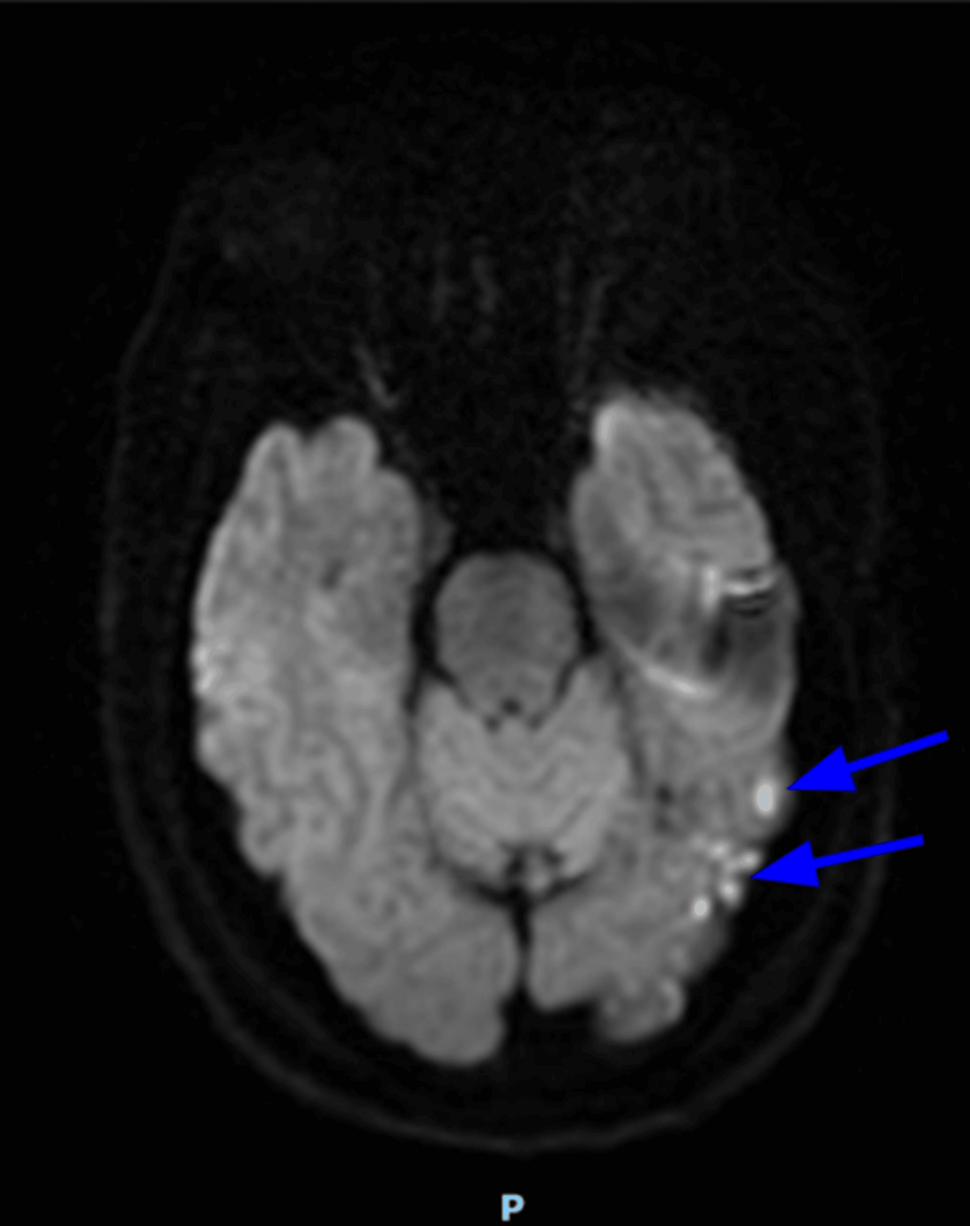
Axial MRI diffusion-weighted image with small foci of enhancement (blue arrows) along the posterior left temporal and left occipital lobes with leptomeningeal enhancement MRI: magnetic resonance imaging
